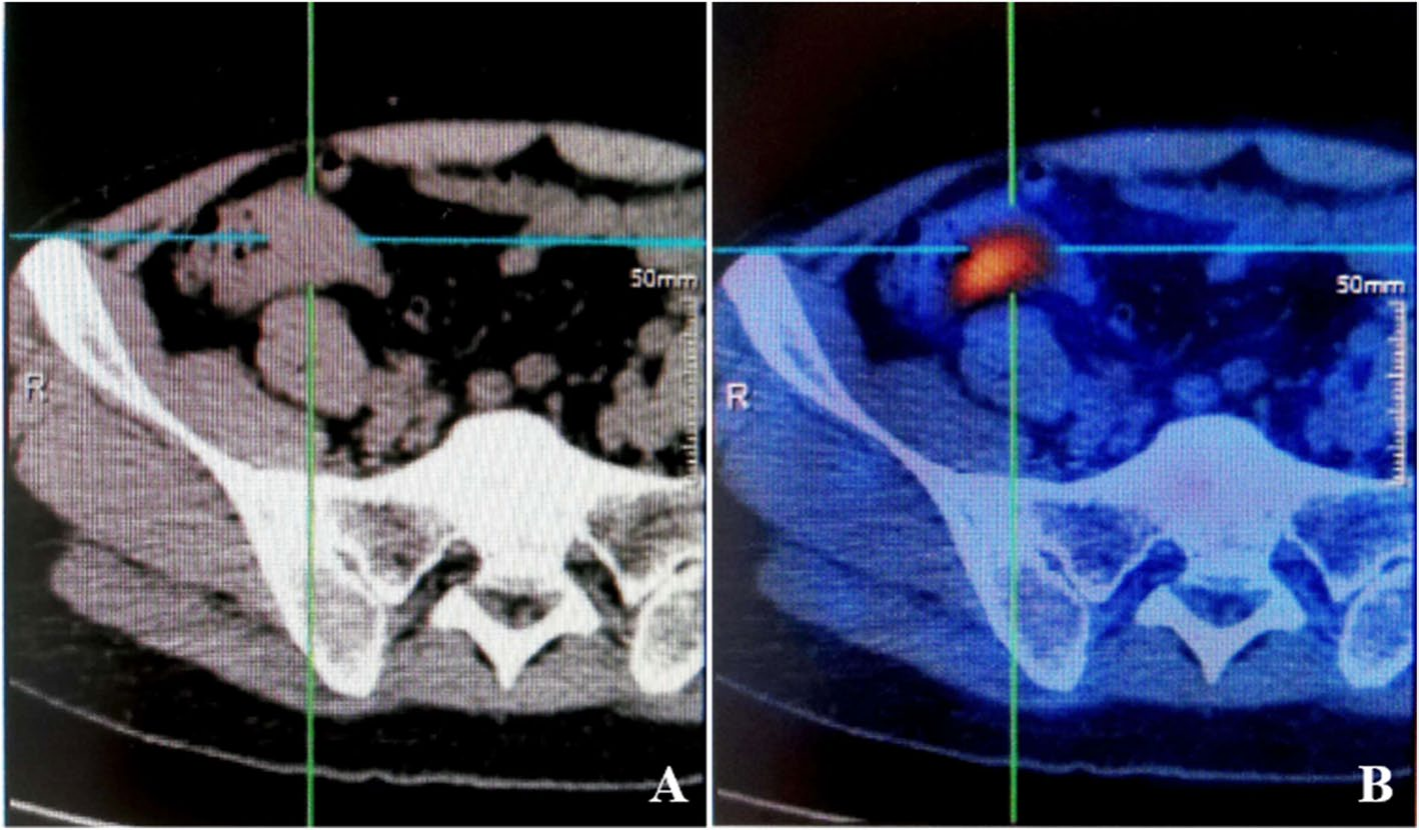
PET/CT findings. A Focal thickening of the cecum wall, about 13 mm. B Increased FDG uptake of the lesion
